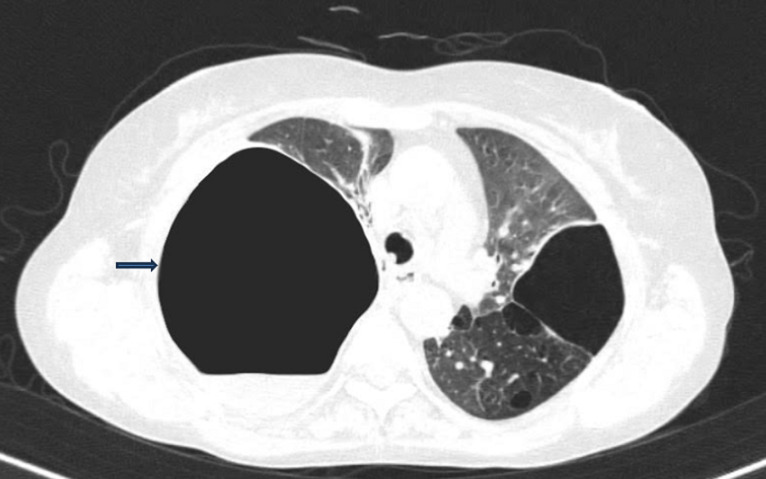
an axial section of computed tomography of lungs showing a giant apical bulla on the right side with air-fluid level inside and compressing surrounding lung parenchyma
